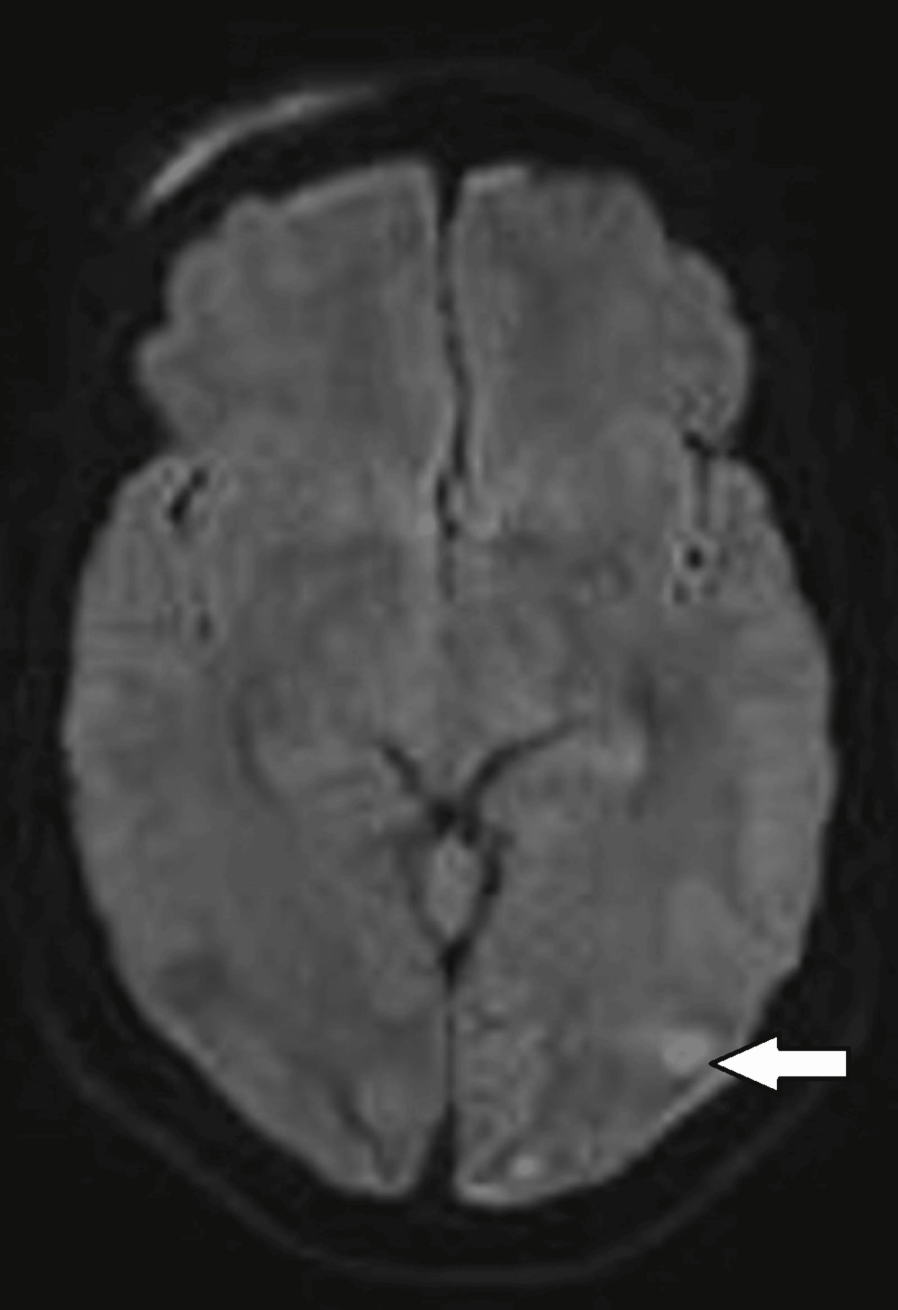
DWI Sequence on Magnetic Resonance Imaging in Case 1 The white arrow represents a diffusion restriction suggesting ischemia DWI: Diffusion weighted imaging
